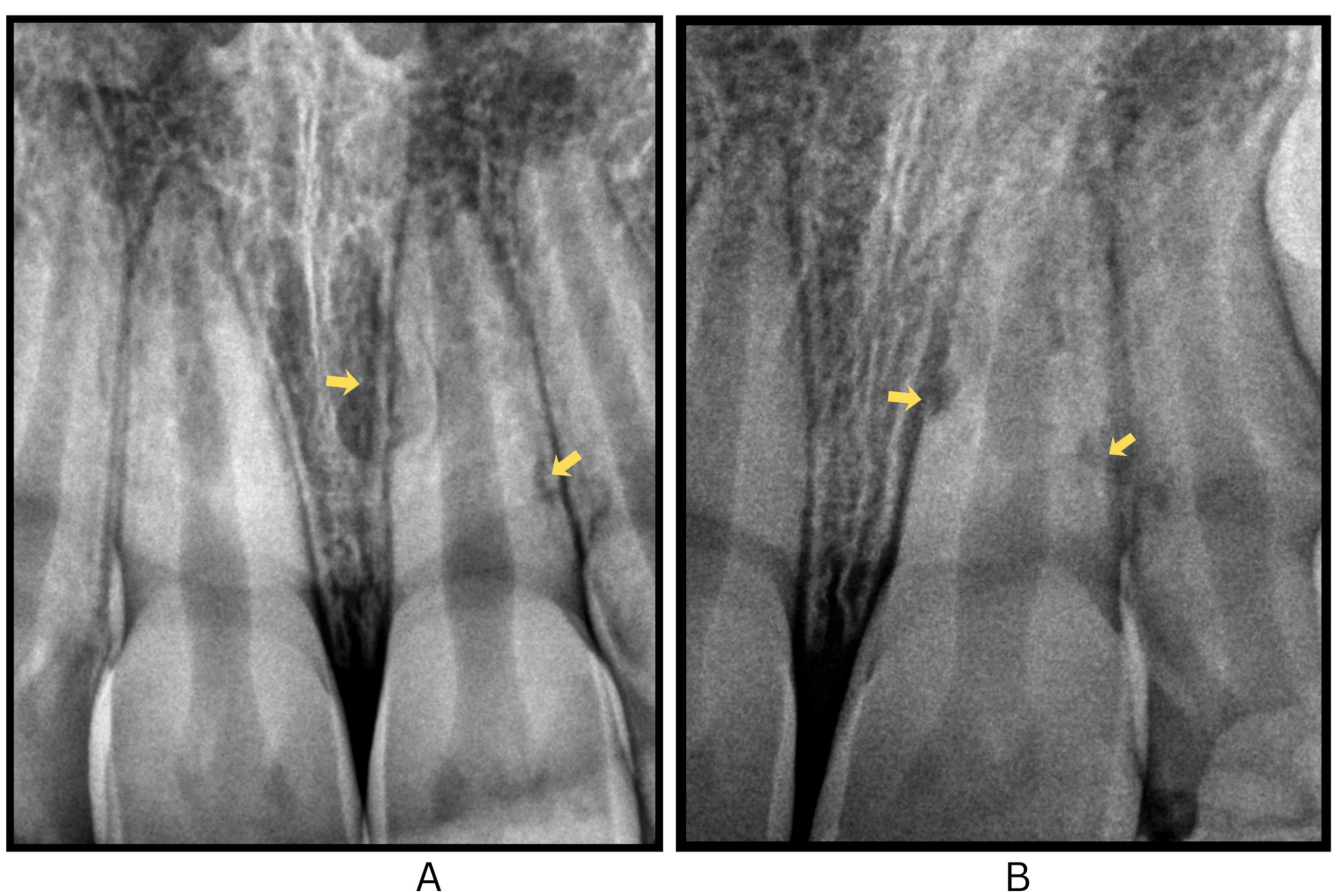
Long-term follow-up periapical radiographs of the replanted maxillary left central incisor. (A) Intraoral periapical radiograph taken one year post-replantation showing stable, non-progressive resorptive changes on the mesial and distal surface (marked by arrows) of tooth #21, with the apex remaining immature. (B) Two-year follow-up radiograph demonstrating tooth #21 with a persistent open apex.
